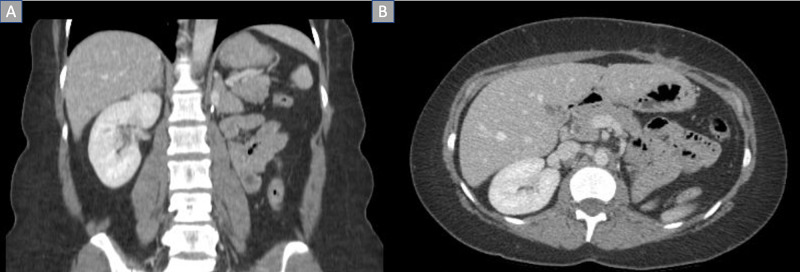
CT abdomen post left radical nephrectomy Surveillance CT abdomen demonstrating left nephrectomy with no residual disease in (A) coronal view and (B) transverse view.
